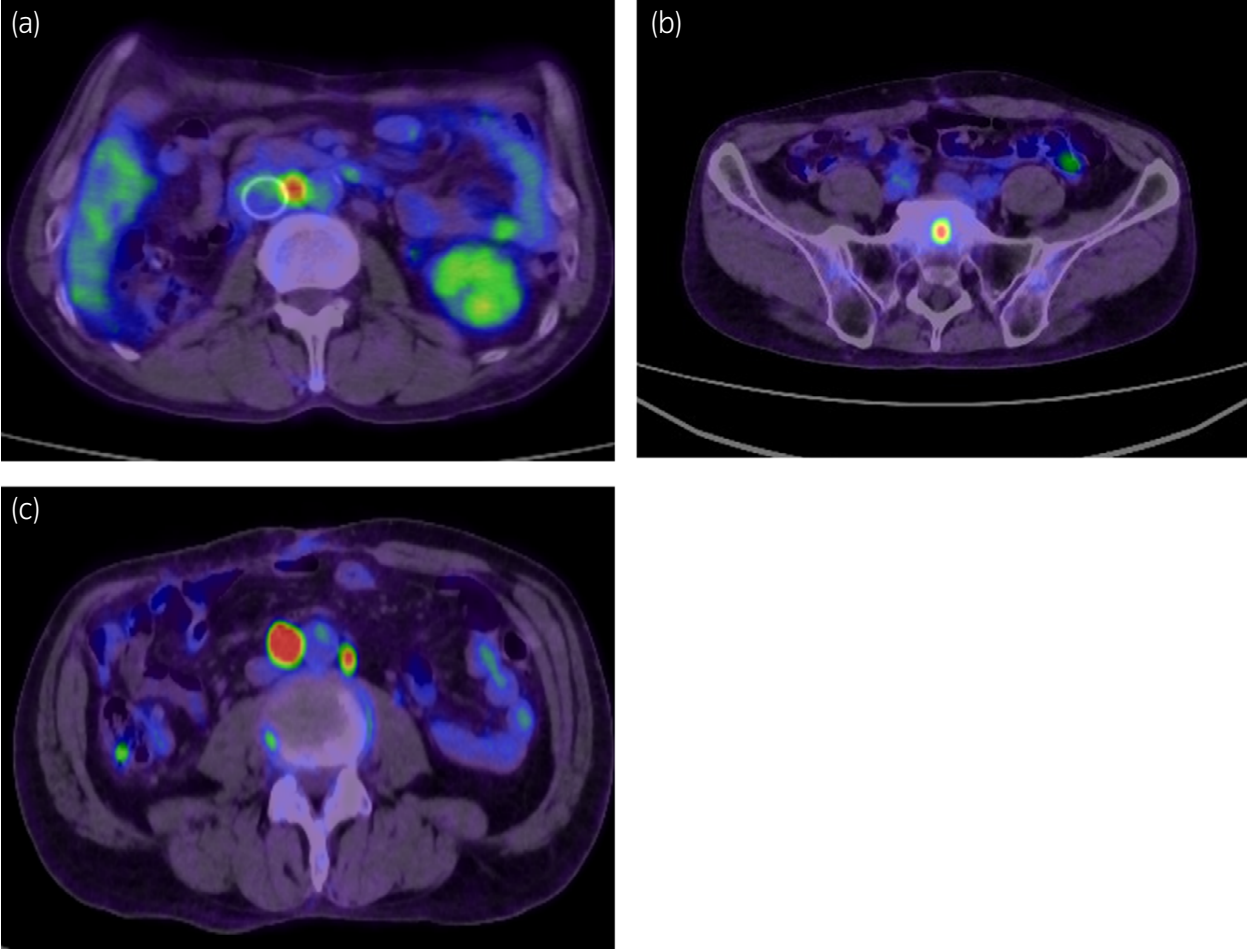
^18^F‐FDG‐PET showed (a) retroperitoneal lymph node metastasis 1 year after the first surgery, and (b) sacrum metastasis and (c) multiple retroperitoneal lymph node metastases 4 months after the second surgery.
